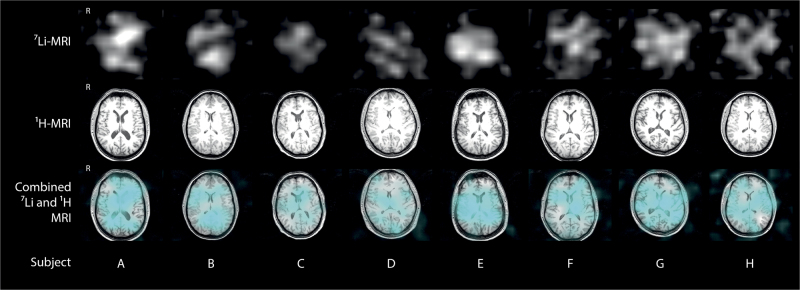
^7^Li b-SSFP MRI in eight subjects shown at the level of the head of the caudate nucleus (upper row), with corresponding ^1^H-MRI axial slices (middle row). The combined images (lower row) show ^7^Li b-SSFP MRI (in cyan) overlaid on ^1^H-MRI images. The distribution of lithium in the brain is heterogeneous in all subjects and varies between subjects
